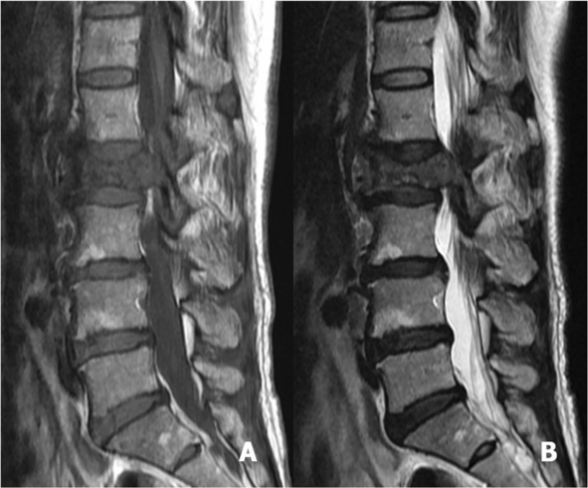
Sagittal T1-weighted MRI (a) and sagittal T2-weighted MRI (b) revealed a pathological fracture at L2, with spinal cord compression
